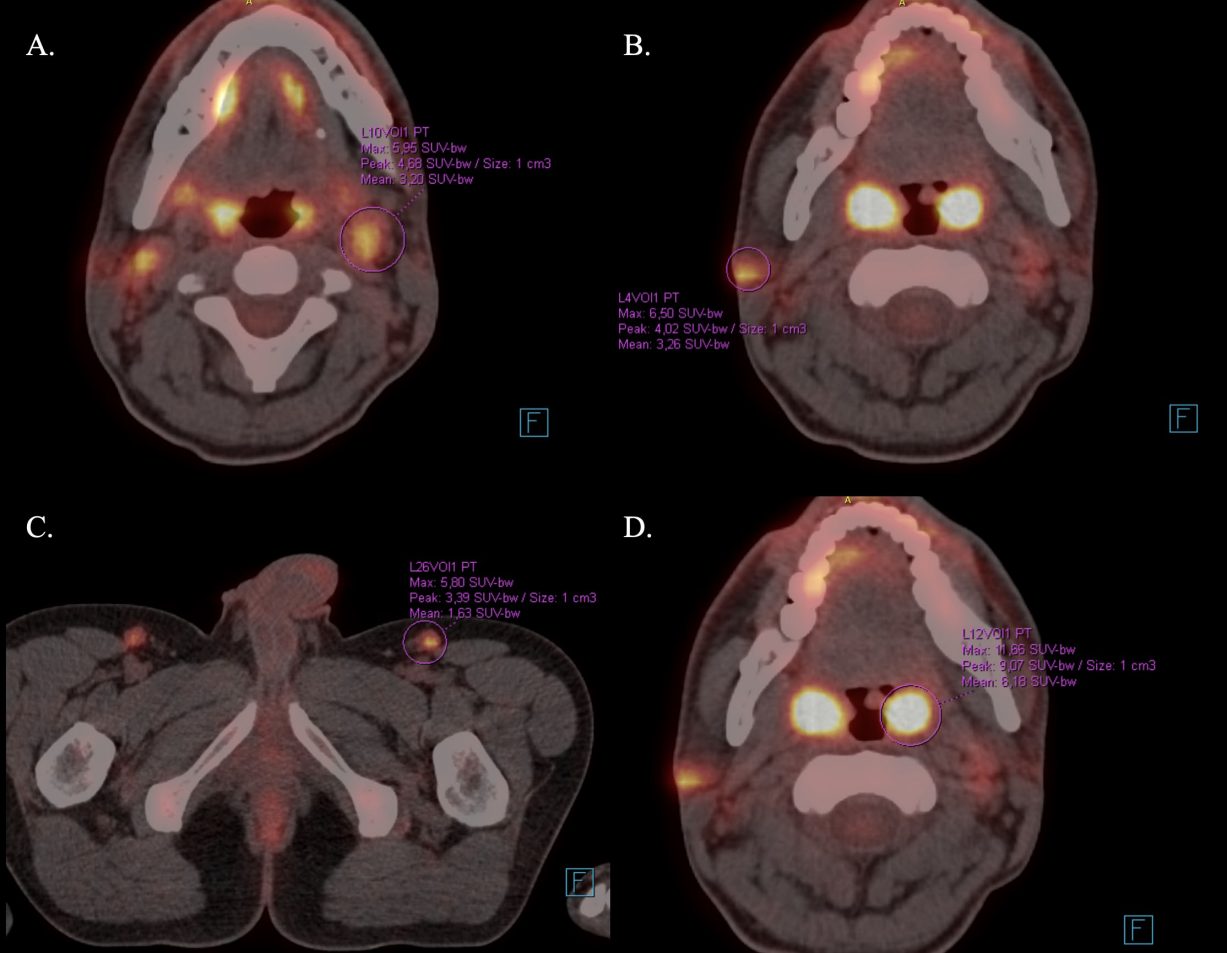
FDG-PET-CT demonstrating diffuse hypermetabolic lymphadenopathy above and below the diaphragm, including bilateral cervical (A), axillary, inguinal (C), and para-aortic lymph nodes (SUVmax up to 6.8), with symmetric tonsillar uptake (D) (SUVmax 11.7) and focal FDG uptake in the left vocal fold (SUVmax 8.2) and skin (B) (SUVmax 6.5). No structural pulmonary lesions were identified. In conjunction with the available results back then, the imaging pattern initially raised strong suspicion of lymphoproliferative disorder.
